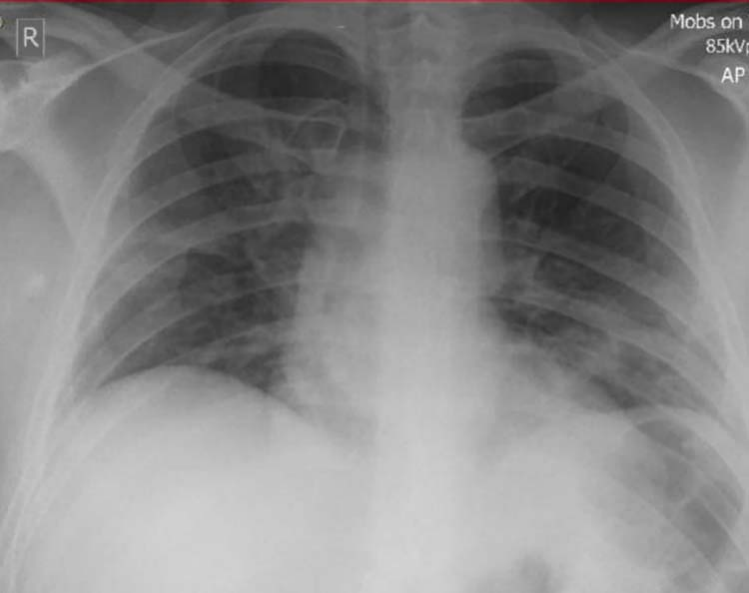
Chest X-Ray of patient on day 2 postadmission (Courtesy of East Suffolk North Essex NHS Foundation Trust Radiology Department).
